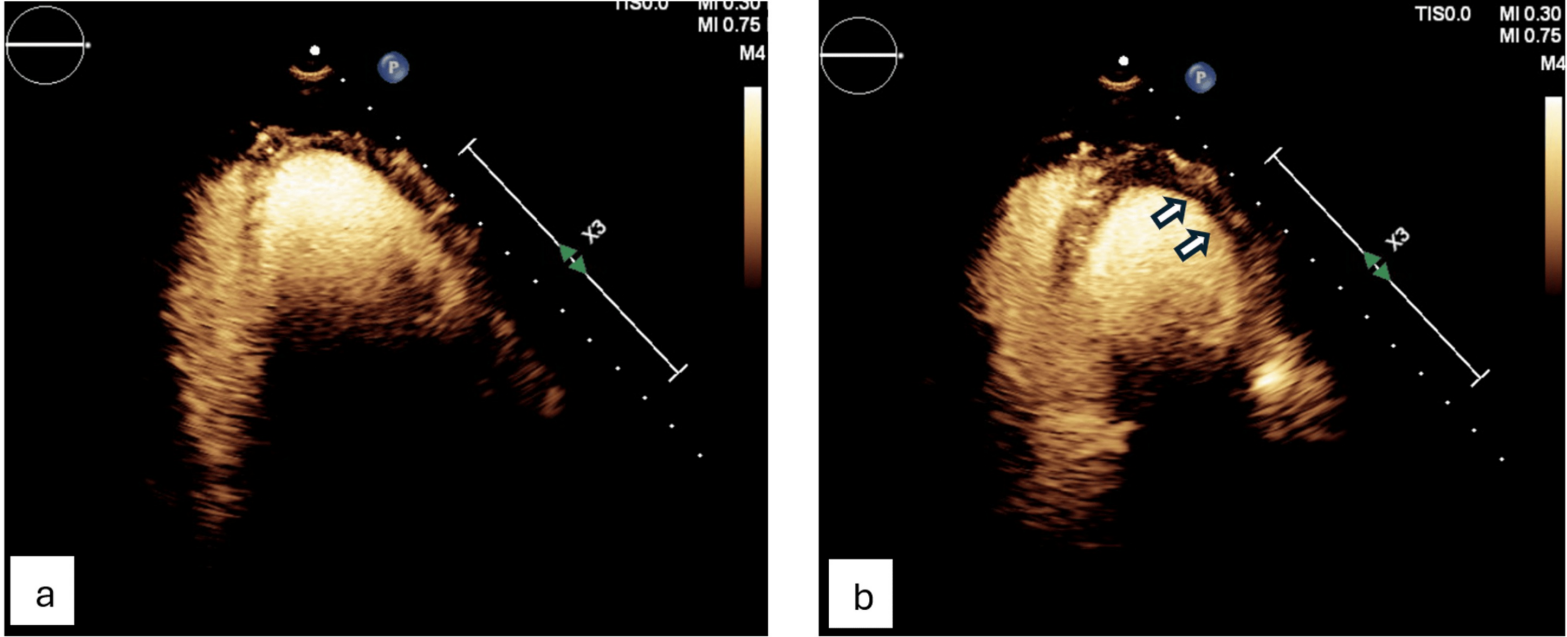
Transthoracic echocardiogram (apical two-chamber view) showing hypokinesis of the anterior wall. (a) Wall motion in diastole. (b) Wall motion in systole.
